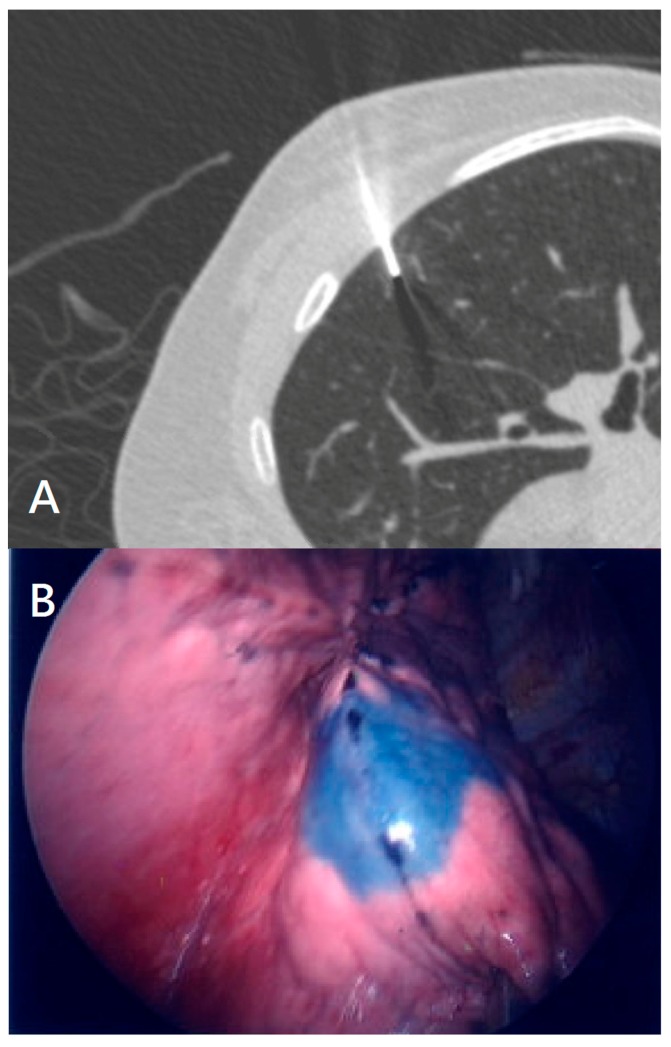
(A) CT-guided localization of fine-needle localization with PBV (Patent Blue Vital); (B) Pleural marking by CT-guided localization method revealed by VATS.
